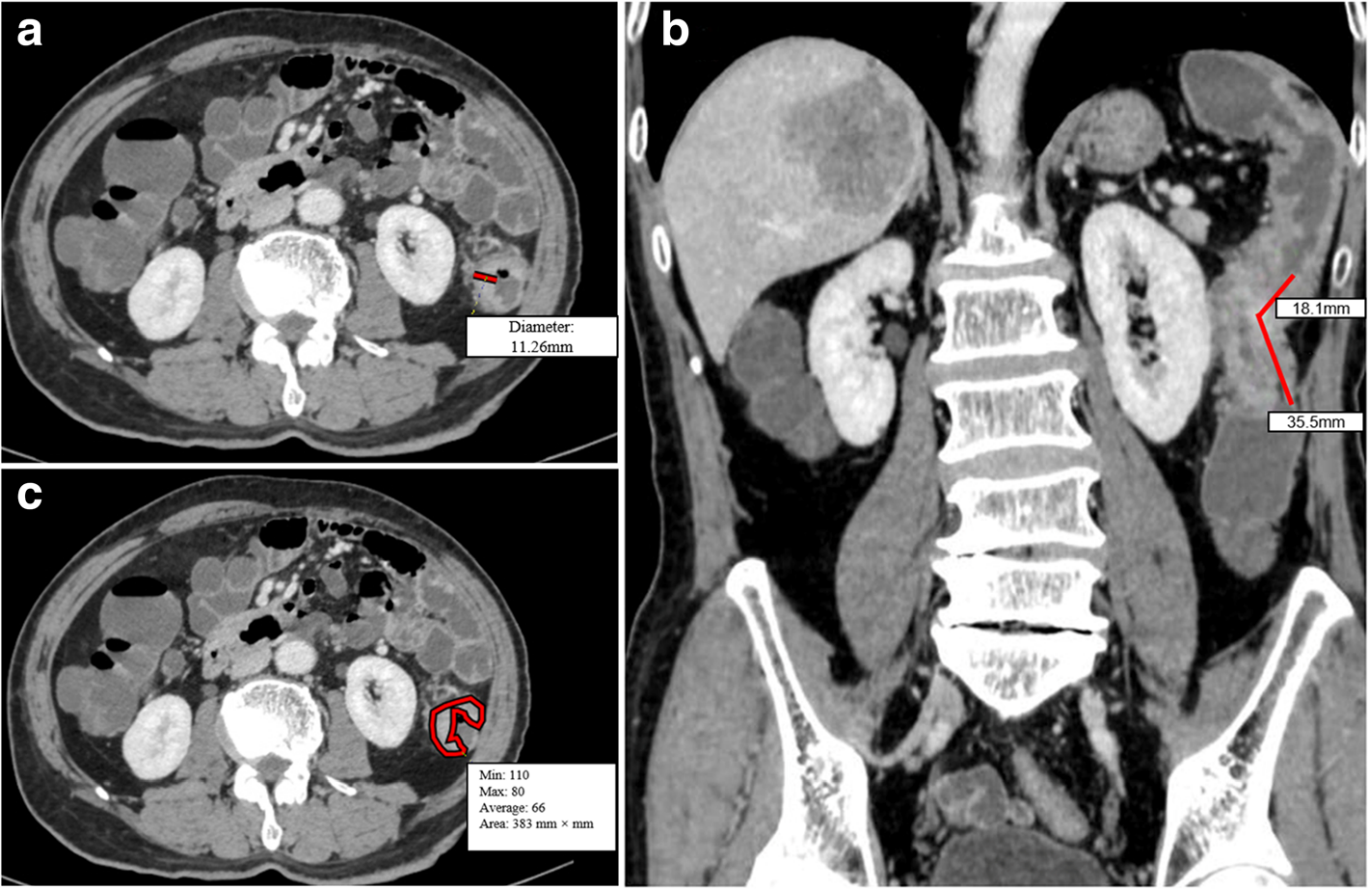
Tumor size measurement using enhanced CT in a 73-year-old man with colon cancer. a Tumor maximum diameter (Tdia) which is perpendicular to the colonic wall. b The reconstructed coronal image to measure tumor length (Tlen) with a broken line. c The manually drawn area along the margin of the tumor, and the value of this area (383 mm^2^) is automatically derived by software together with minimal, maximal, and average CT attenuation (in Hounsfield units)
